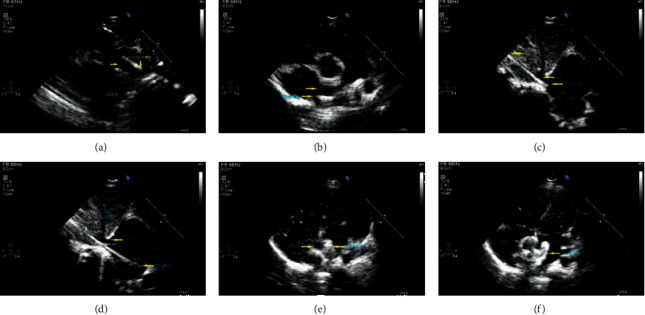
Optimization of echo views: (a) subcostal section shows the guidewire going through the inferior vena cava; (b) parasternal short-axis section of the aorta shows the catheter going through the ASD into the left atrium; (c) subcostal biatrial section shows the sheath going through the inferior vena cava; (d) subcostal biatrial section shows the sheath going through the ASD into the left atrium; (e) parasternal four-chamber view shows release of the left disc of the device; (f) apical four-chamber view shows release of the right disc of the device.
